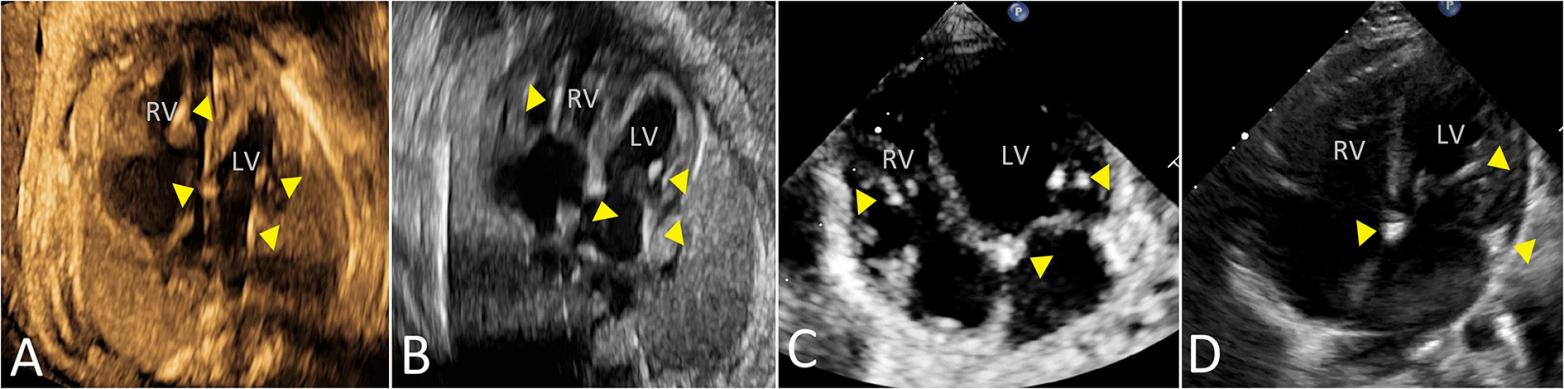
The echocardiographic features of isolated mild EFE in a fetus due to maternal anti-Ro and anti-La antibodies. Triangles mark the areas of mild EFE in four-chamber view [(A) 30 gestational weeks; (B) 33 gestational weeks; (C) 1 month; (D) 1 year]. LV, left ventricle; RV, right ventricle.
